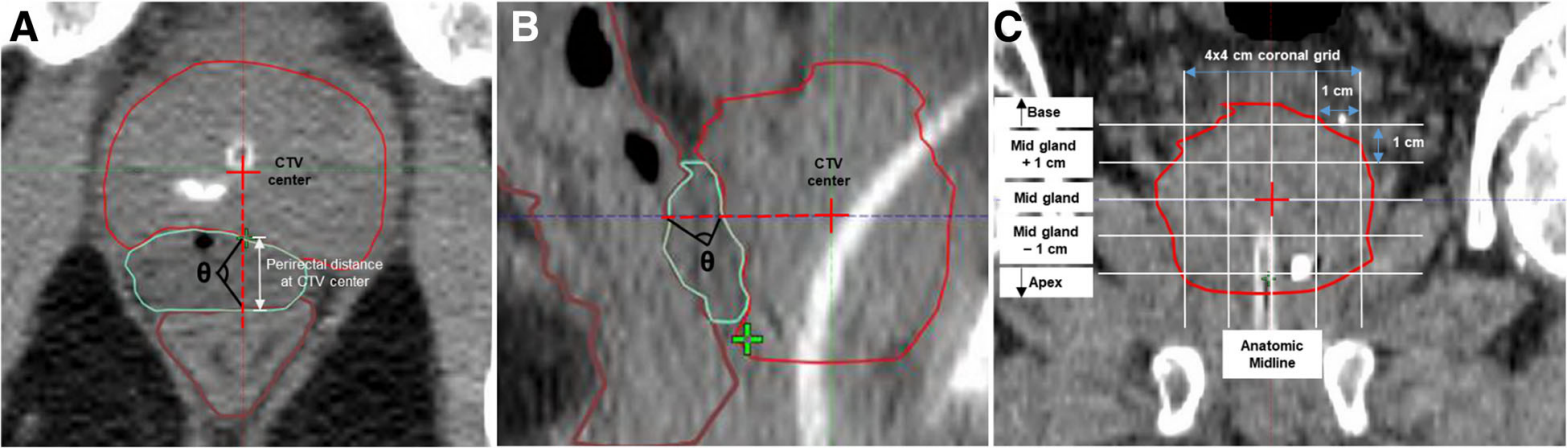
Measurement of a perirectal distance at CTV center on axial CT image (a), and θ in 3-dimensional space on axial and sagittal CT images (a, b). The perirectal distance is measured at each vertex in a 4 × 4 cm coronal grid centered on the prostate midgland, at midline to generate a perirectal space map comprising up to 25 perirectal distance measurements for each patient (c)
